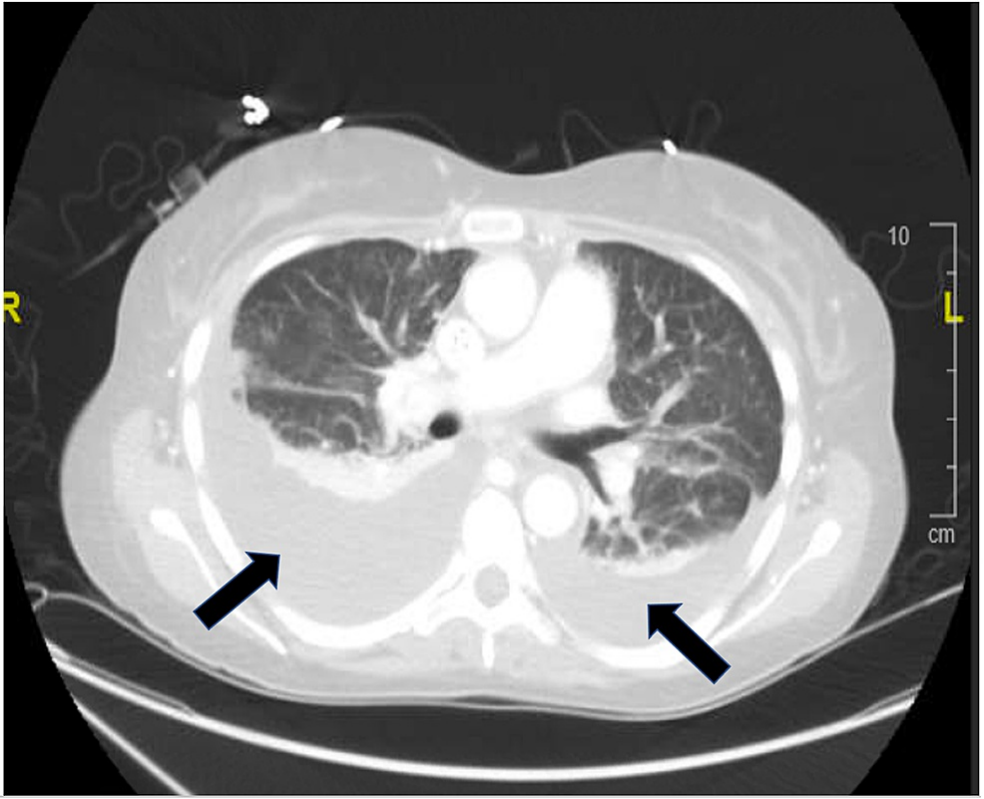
CT chest, transverse section, demonstrating bilateral pleural effusions (black arrows, right larger than left).
